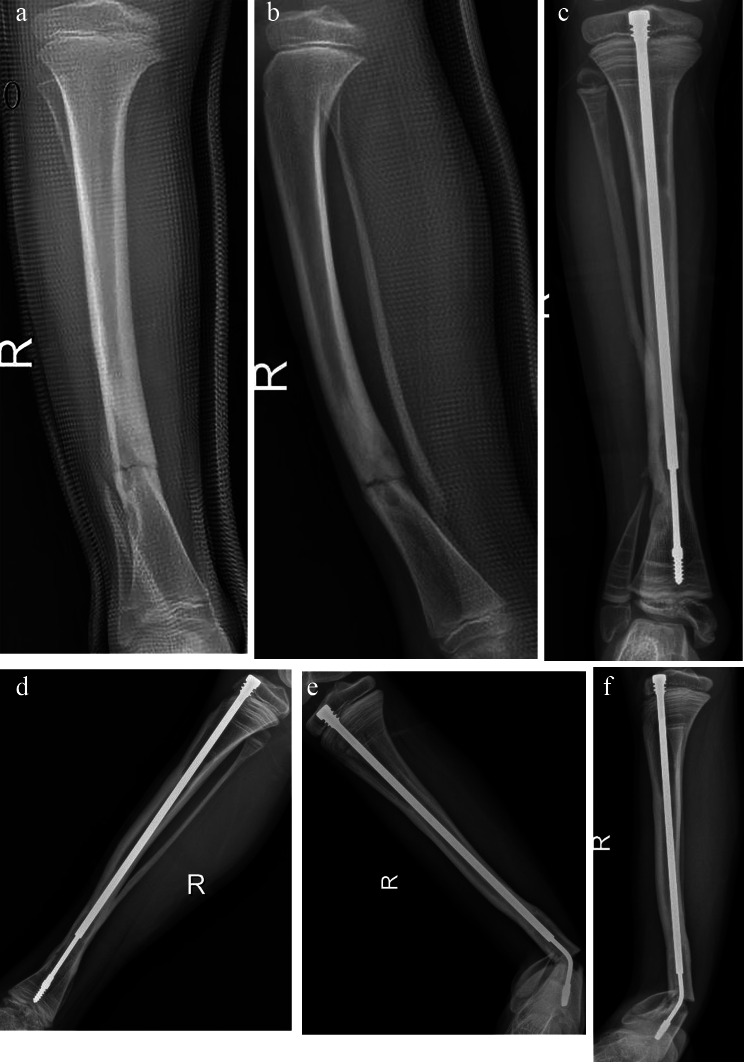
A and B: X-rays of the tibia of a 9-year-old male child with CPT before operation; C and D: Anteroposterior and lateral radiographs in two years and three months after CPT showed distal displacement of the intramedullary rod. E and F: Anteroposterior and lateral radiographs at 2 years and 10 months after CPT showed re-fracture with bent inner core
